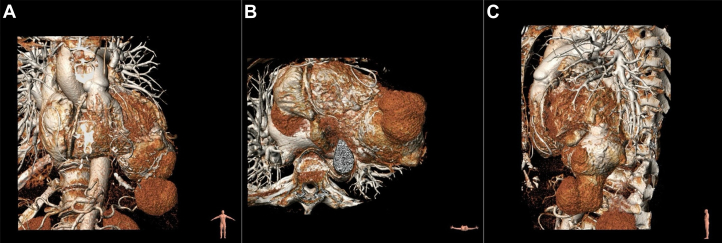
Computed Tomography 3-Dimensional Volume Rendering Reconstruction (A) Coronal view. (B) Axial view. (C) Sagittal view.
